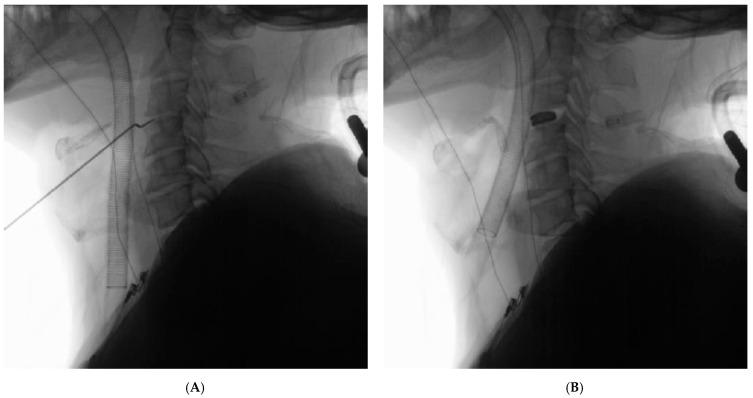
Intraoperative fluoroscopic lateral radiographs demonstrating C3–4 anterior cervical discectomy and fusion (ACDF). (A) Intraoperative lateral fluoroscopic radiograph obtained during level localization and alignment assessment prior to discectomy, demonstrating accurate identification of the C3–4 disk space. The endotracheal tube is visible anteriorly, reflecting airway instrumentation in the context of limited cervical mobility. (B) Intraoperative lateral fluoroscopic radiograph following placement of the interbody cage, confirming appropriate implant positioning, restoration of disc height, and satisfactory sagittal alignment at the operative level, with no evidence of malposition or instability.
